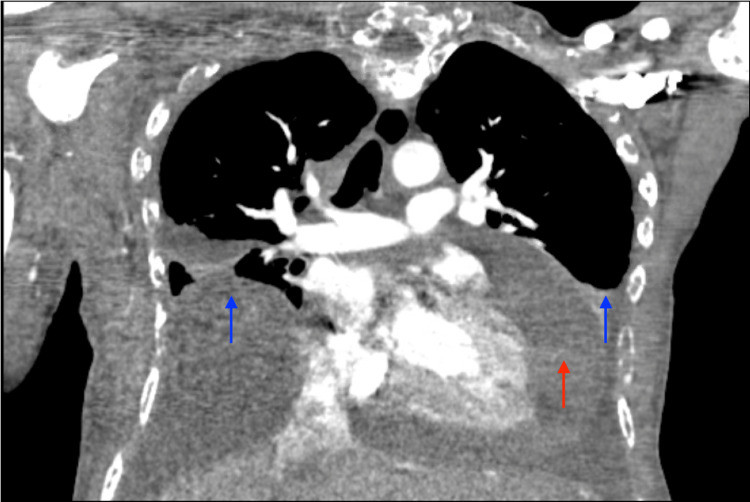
CT scan of the chest in coronal view demonstrates a large pericardial effusion (red arrow) and bilateral pleural effusions (blue arrows).
